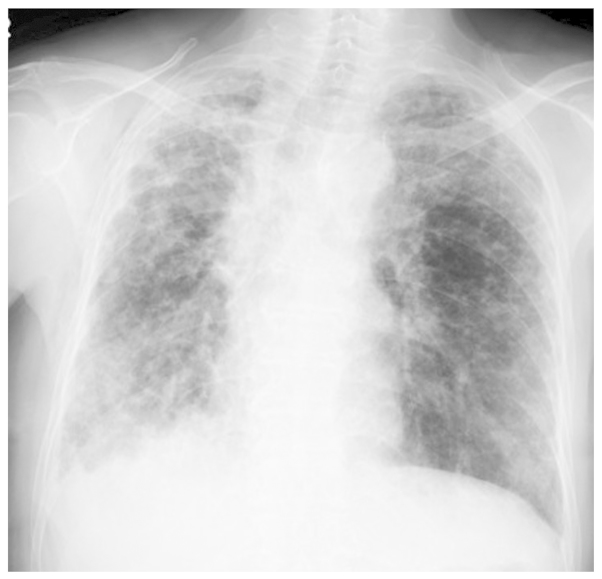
Chest radiograph 8 months after the autologous blood-patch pleurodesis shows no recurrence of pneumothorax.
